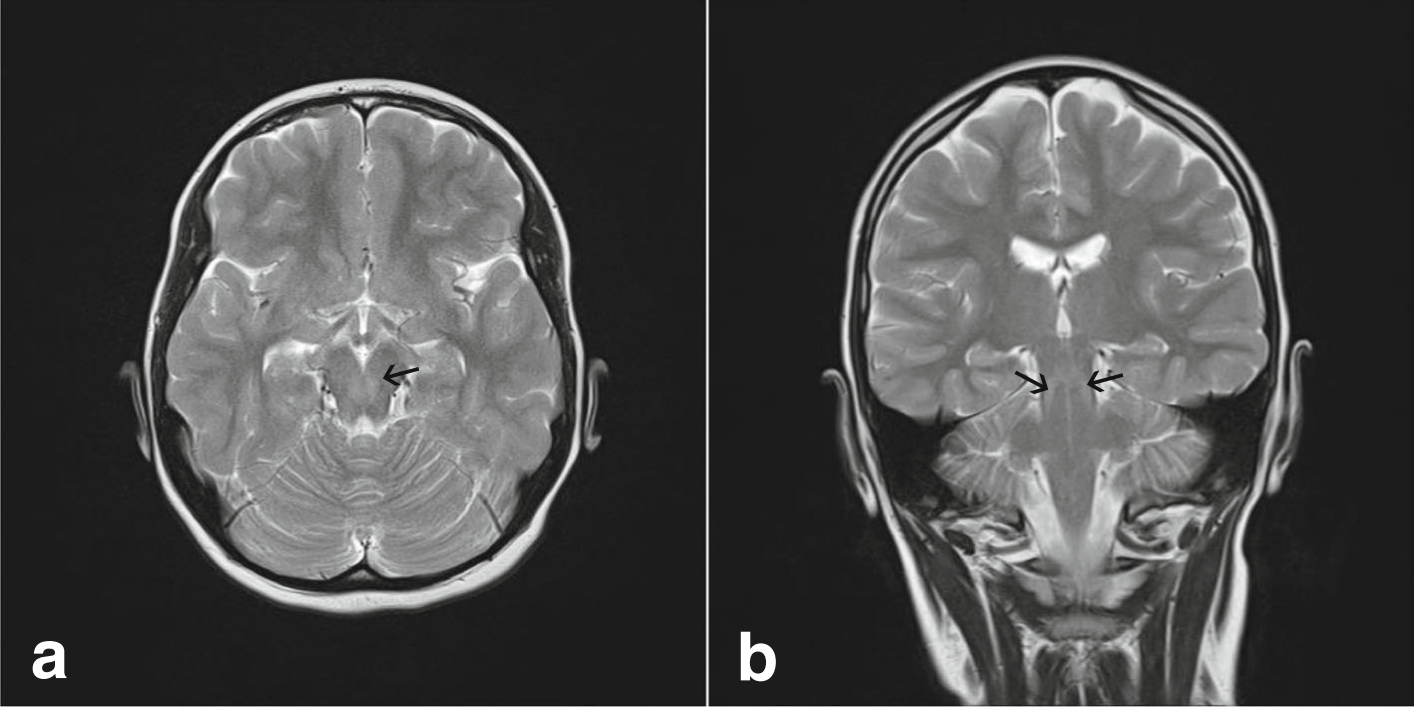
Magnetic resonance imaging scans from patient with PEX10 defect (12 years of age). The magnetic resonance imaging scan shows: a axial T2-weighted imaging and b coronal T2-weighted imaging revealing a central lesion in the mesencephalon (*arrows*), from the nucleus ruber dorsocaudally down to the cerebellar peduncles
